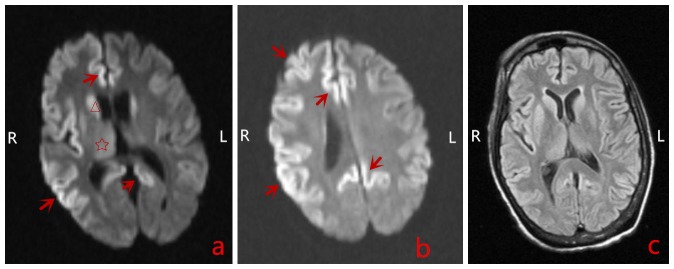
Brain magnetic resonance imaging (MRI) study a) Diffusion-weighted imaging (DWI) showed increased abnormal signal intensities with restricted diffusion in the cerebral cortex bilaterally (arrows, Right > Left), basal ganglia (triangle), and thalami (asterisk); b) DWI showed abnormal hyperintensities with restricted diffusion in the bilateral cerebral cortex (arrows, Right > Left); c) Fluid-attenuated inversion recovery imaging: relatively normal brain MRI imaging.
